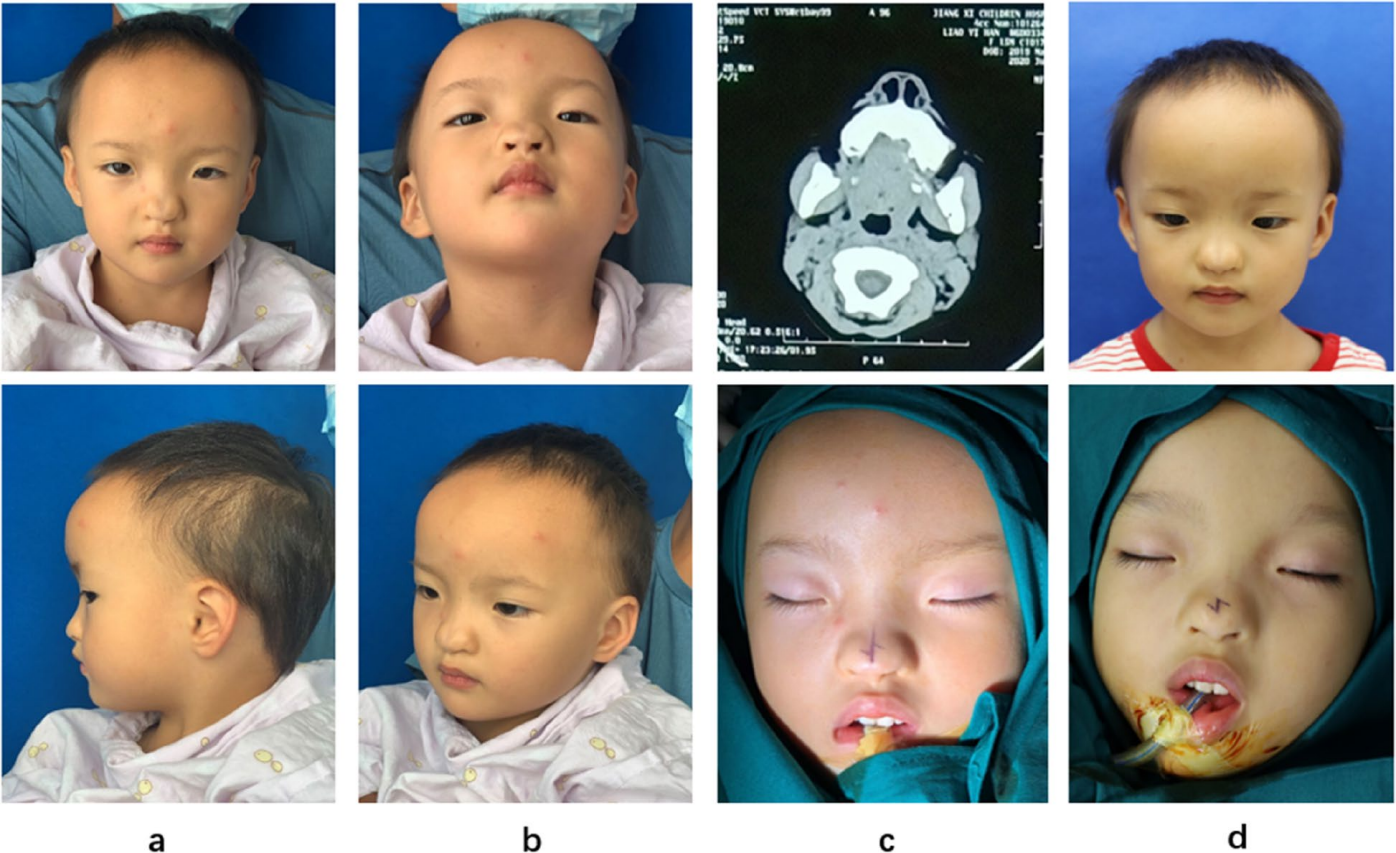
The twin sister photos before the surgery, CT scan with mild bifid nose and the intraoperative marking lines of the incision are shown. a-c The elder sister. d The younger sister
